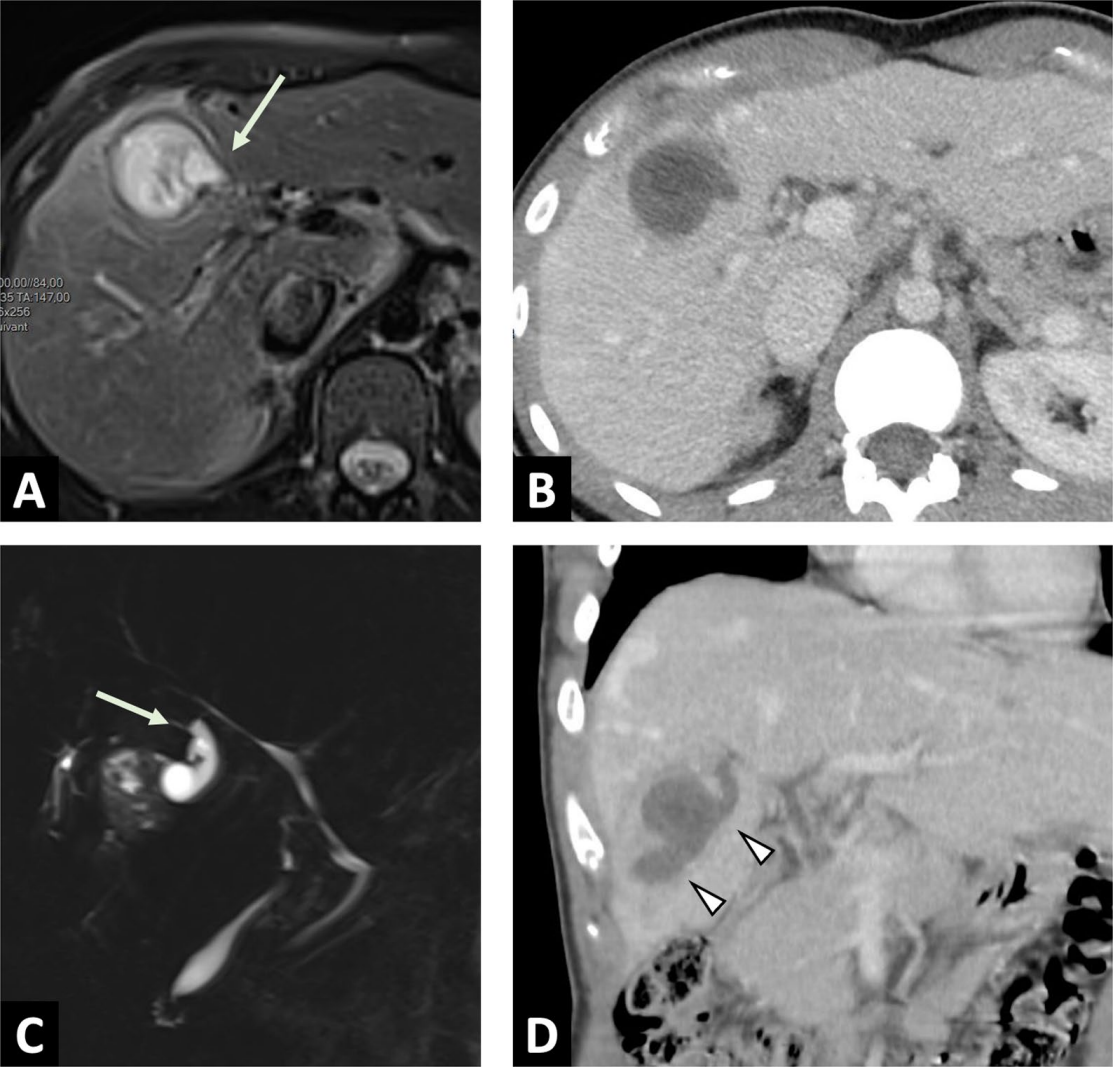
Cystic echinococcosis lesion CE3b of the right liver lobe in a 44-year-old man. A T2-weighted MR image in the axial plane: loss of continuity of the cyst wall and visualization of daughter cyst into the biliary duct (arrow). B Corresponding images on CT obtained during the portal phase of enhancement. C MRCP in the coronal plane: direct communication between the cyst and the biliary duct (arrow). D Corresponding images on CT obtained during the portal phase of enhancement: direct communication between the cyst and the biliary duct (arrowheads)
